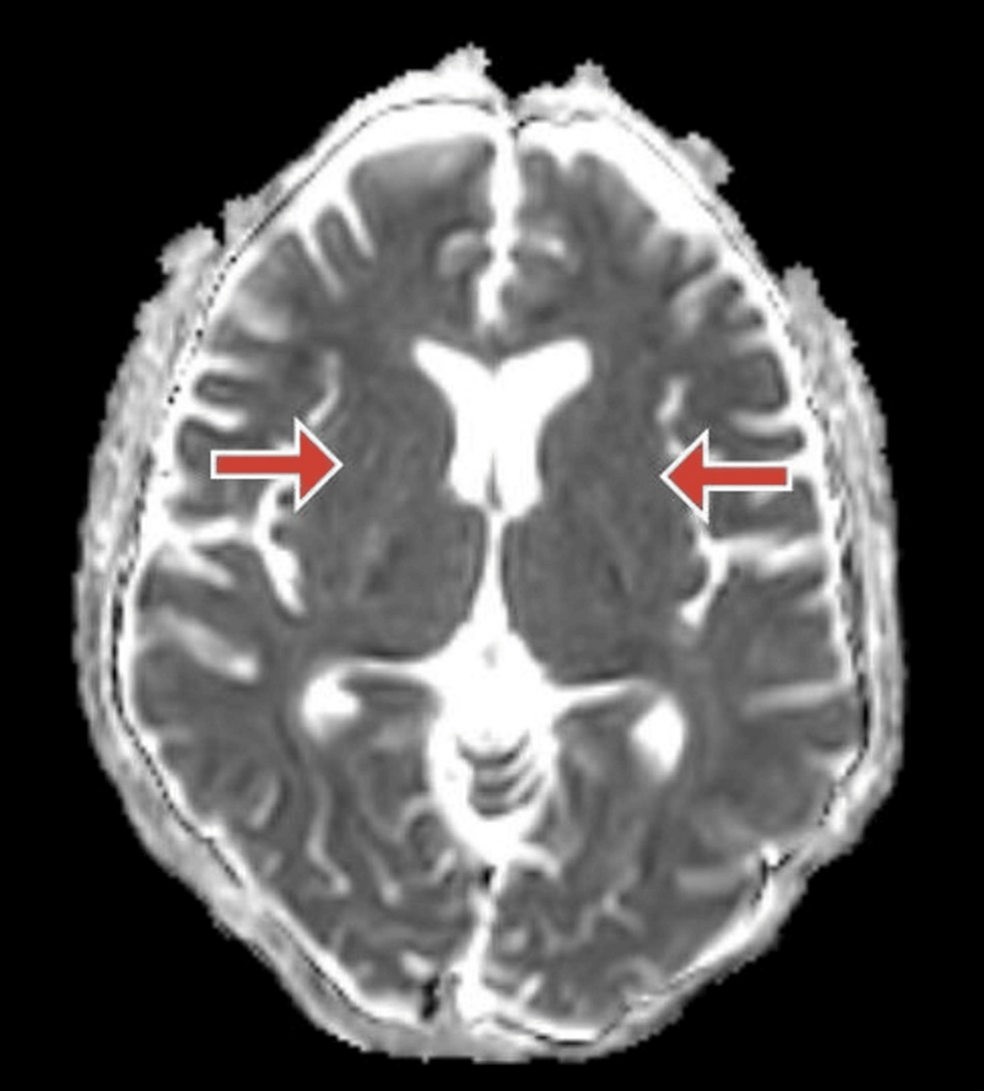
Magnetic resonance imaging apparent diffusion coefficient (MRI-ADC) section of the brain MRI-ADC section of the brain showing restricted diffusion in bilateral basal ganglia.
